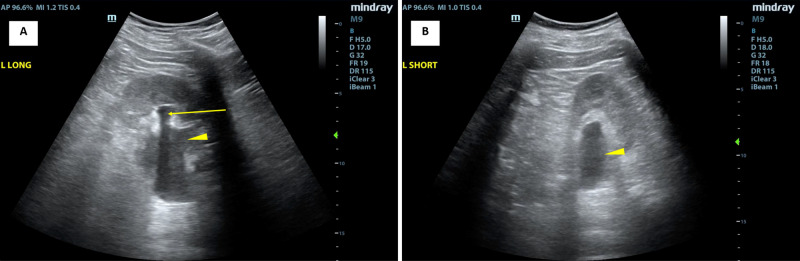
Left-sided renal ultrasound images The left-sided renal ultrasound image shown in the long axis view (A) demonstrates moderate hydronephrosis (marked by the arrow) and hydroureter (marked by the arrowhead). The short-axis view shows hydroureter.
